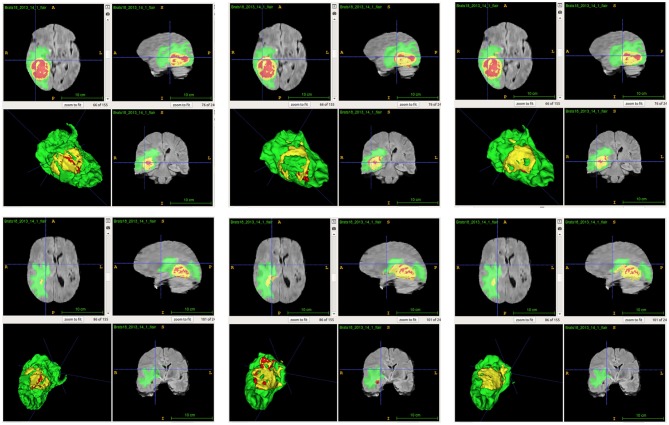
Examples of predictions from single model with different inputs. Top row shows the predictions from DeepMedic, and bottom row shows the predictions from 3D U-Net (from left to right: ground-truth lesions, prediction from single model, and prediction from single model with additional brain parcellation masks.) Red: enhancing tumor, yellow: necrosis & non-enhancing tumor, and green: edema. ITK-SNAP (Fedorov et al., 2012) is used to visualize the MR images and lesion masks.
